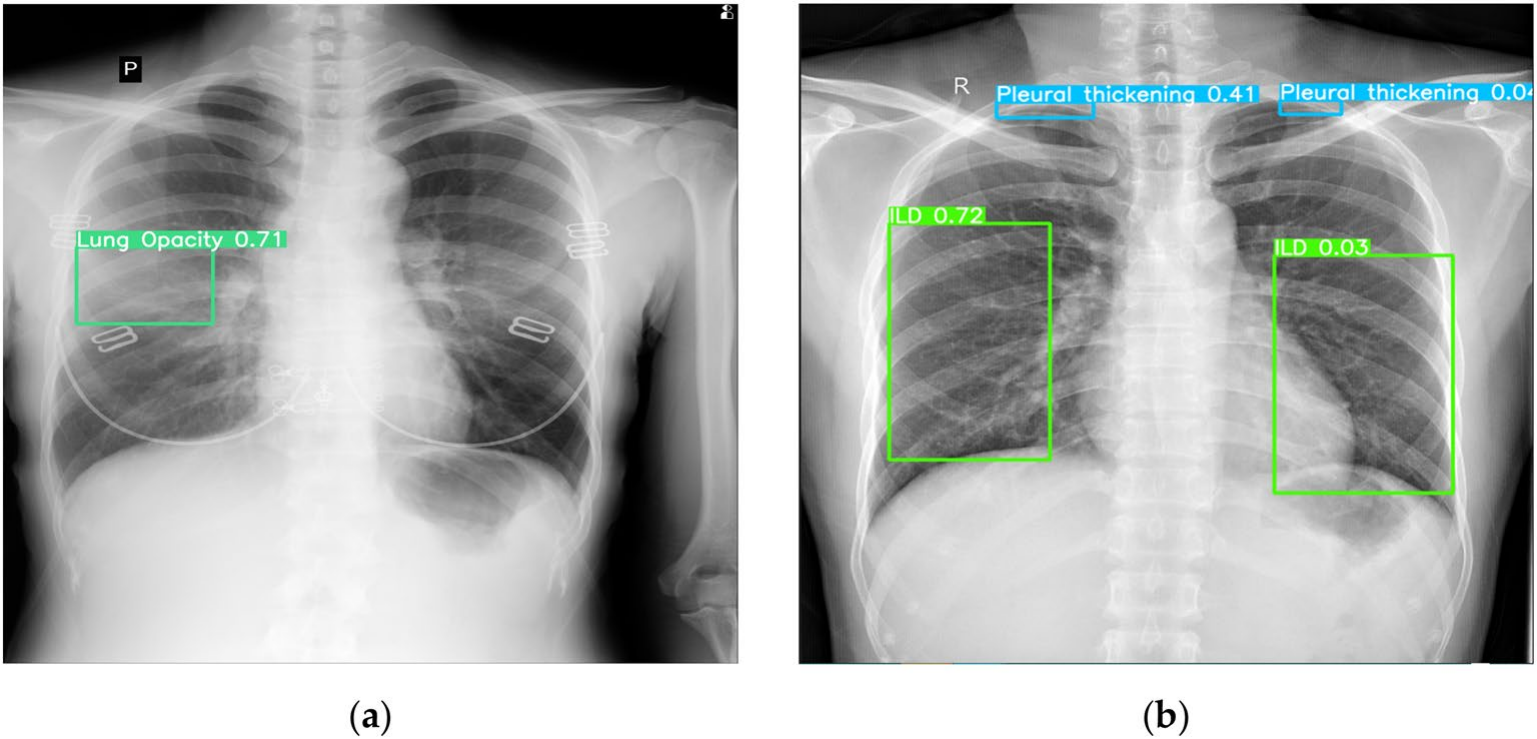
Prediction samples from the deployed model: (a) Prediction of the patient scan showing the presence of Lung opacity; (b) Prediction from another chest radiograph exam that shows the existence of four findings and pathologies: Interstitial lung disease (ILD), Pleural thickening.
